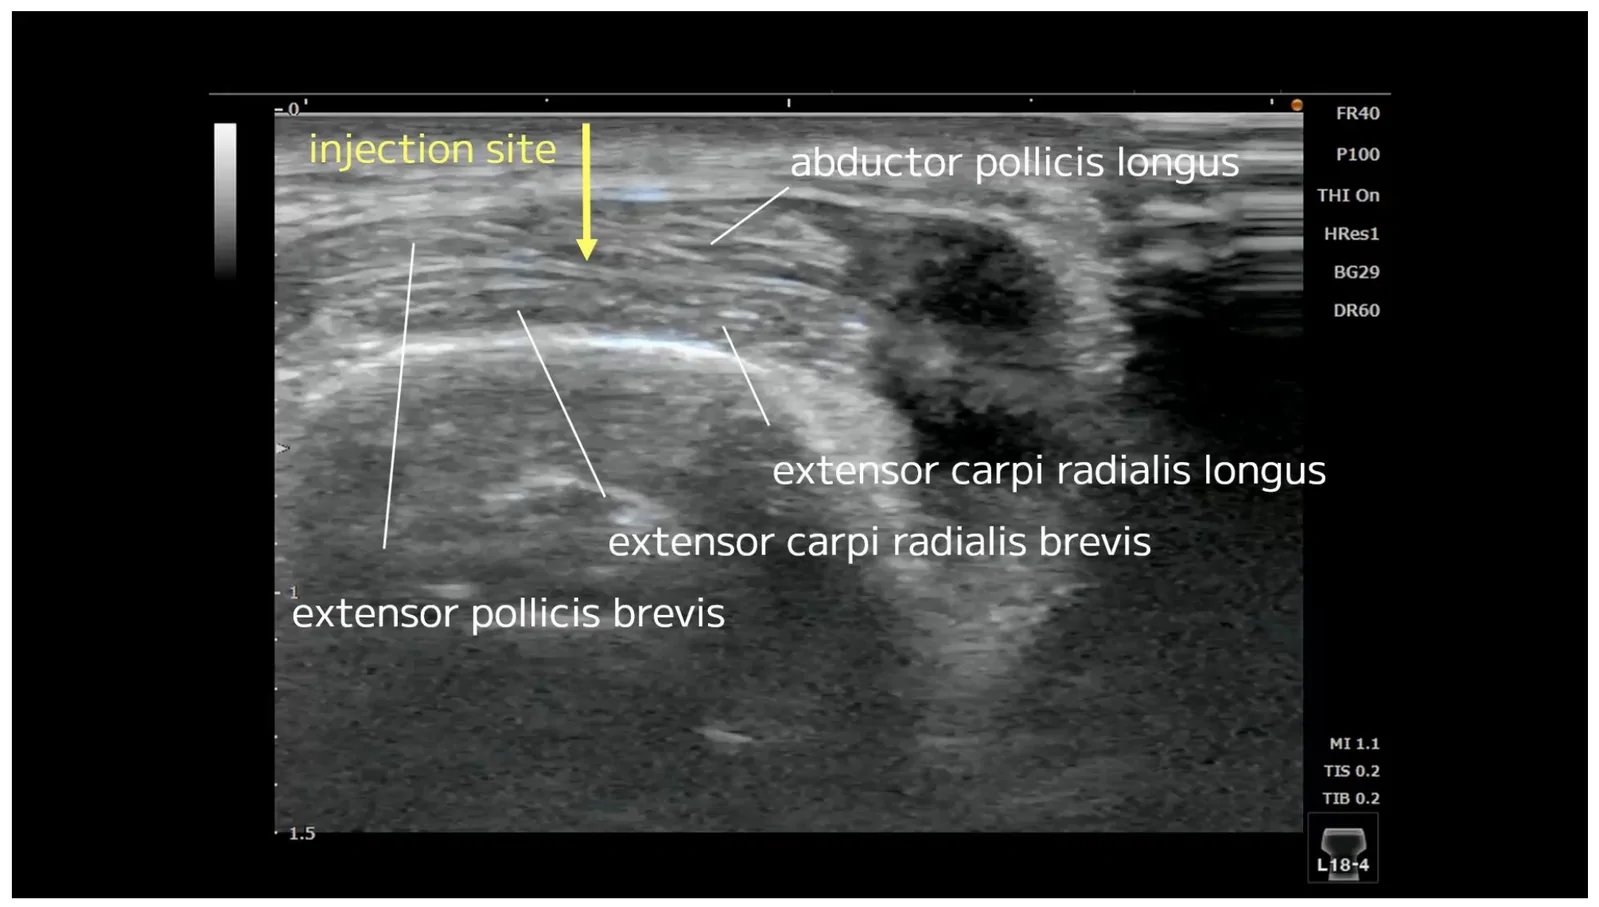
Point 3—first–second compartment intersection: ultrasound-guided fascia hydrorelease; yellow arrow indicates the injection site.
